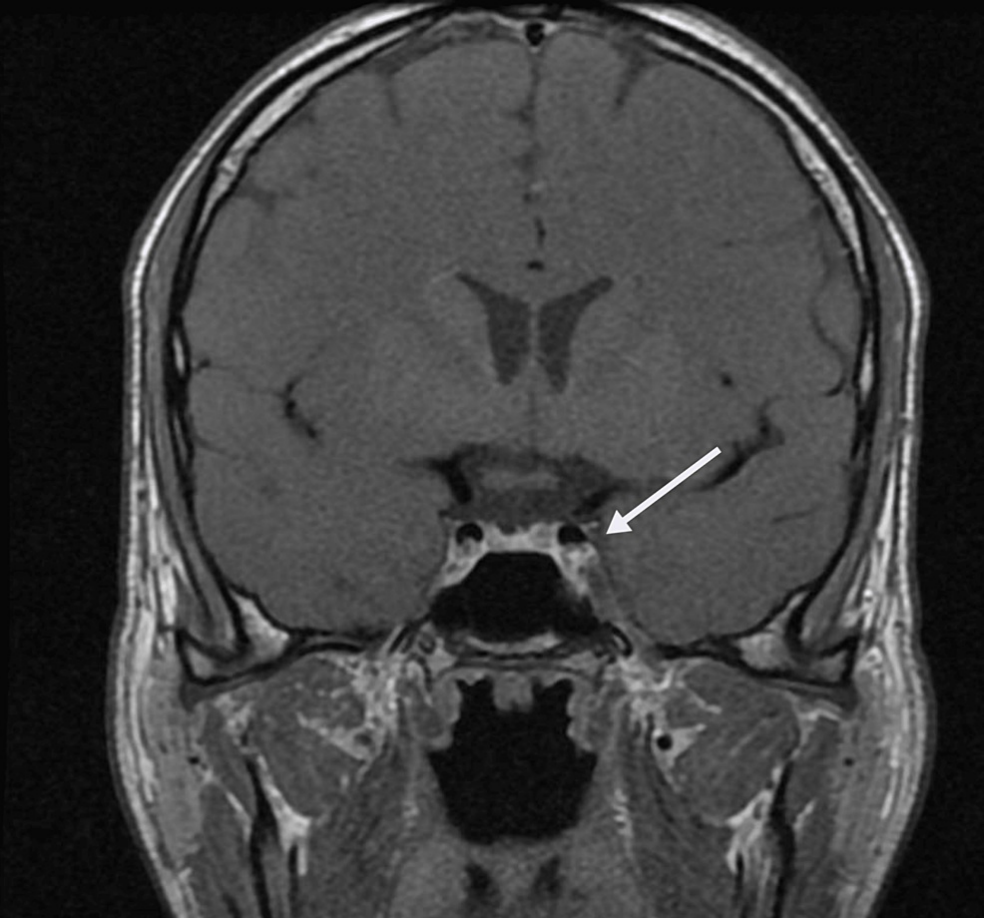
Magnetic resonance imaging (MRI) of head obtained during hospital course
